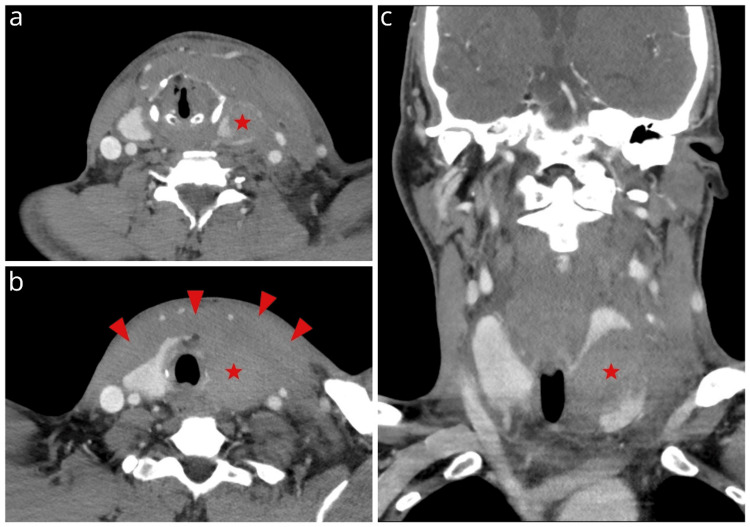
Three-day contrast-enhanced computed tomography (CECT) follow-up with axial images (a, b) and coronal reconstruction (c) The images indicate the stability of the haematoma size (star) and diffuse hyperdense haemorrhagic infiltration of the superficial lower cervical structures (arrowheads).
